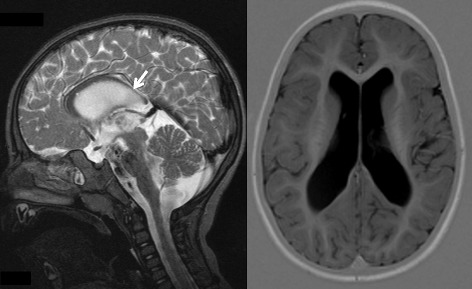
Brain MRI of the proband at age 2 years and 8 months. Note the normal cortex, temporo-parietal subcortical atrophy bilaterally, enlarged lateral ventricles, the thin corpus callosum predominantly in its caudal portion (*arrow*)
